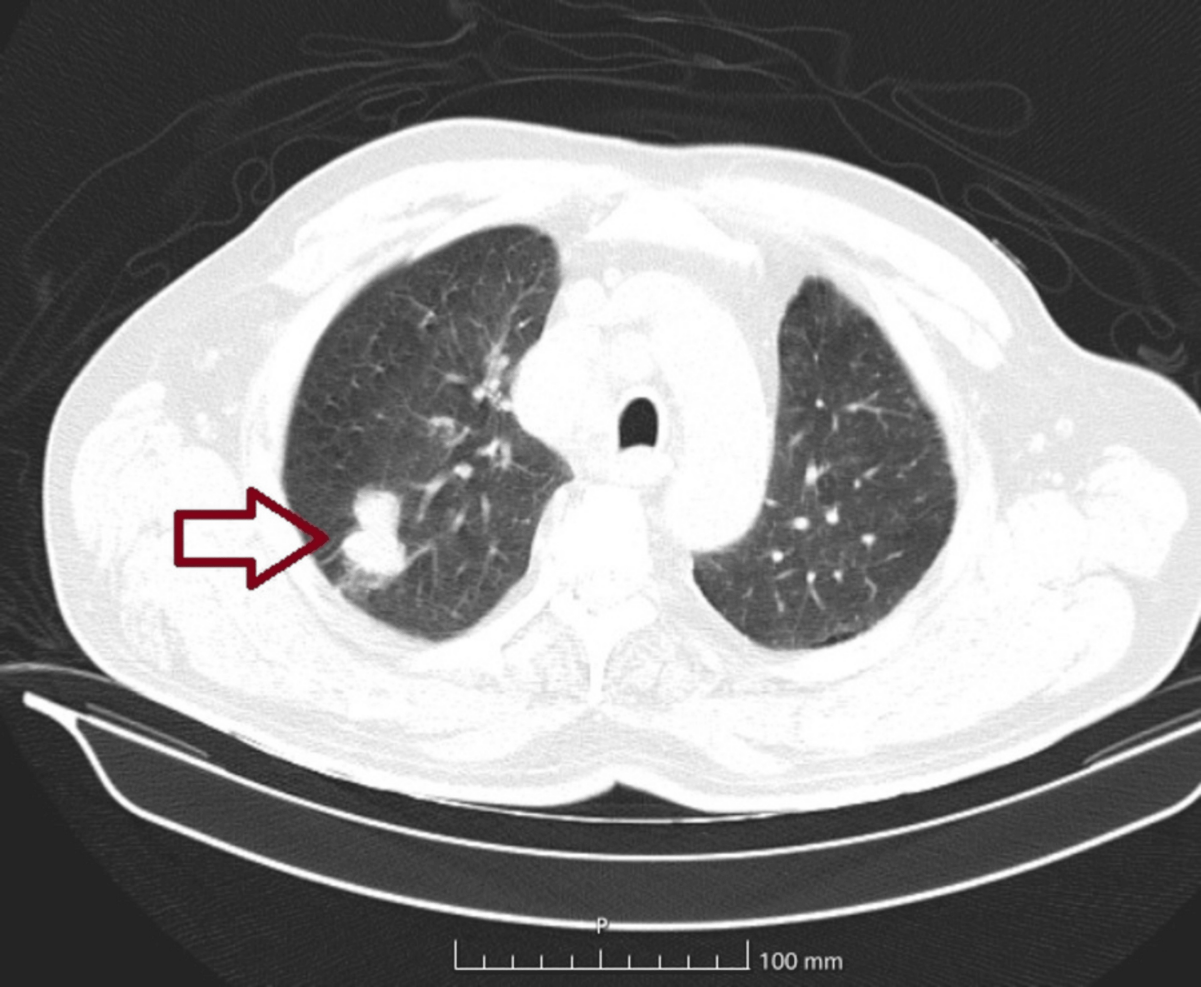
CT scan with a pulmonary mass in the right upper lobe.
